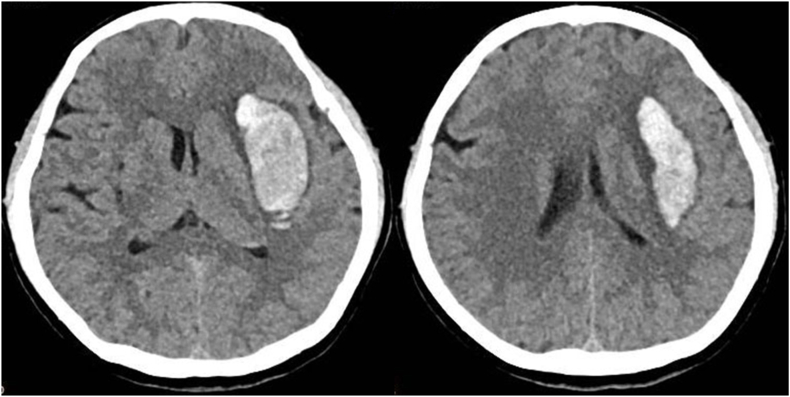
The preoperative CT of case 1.
